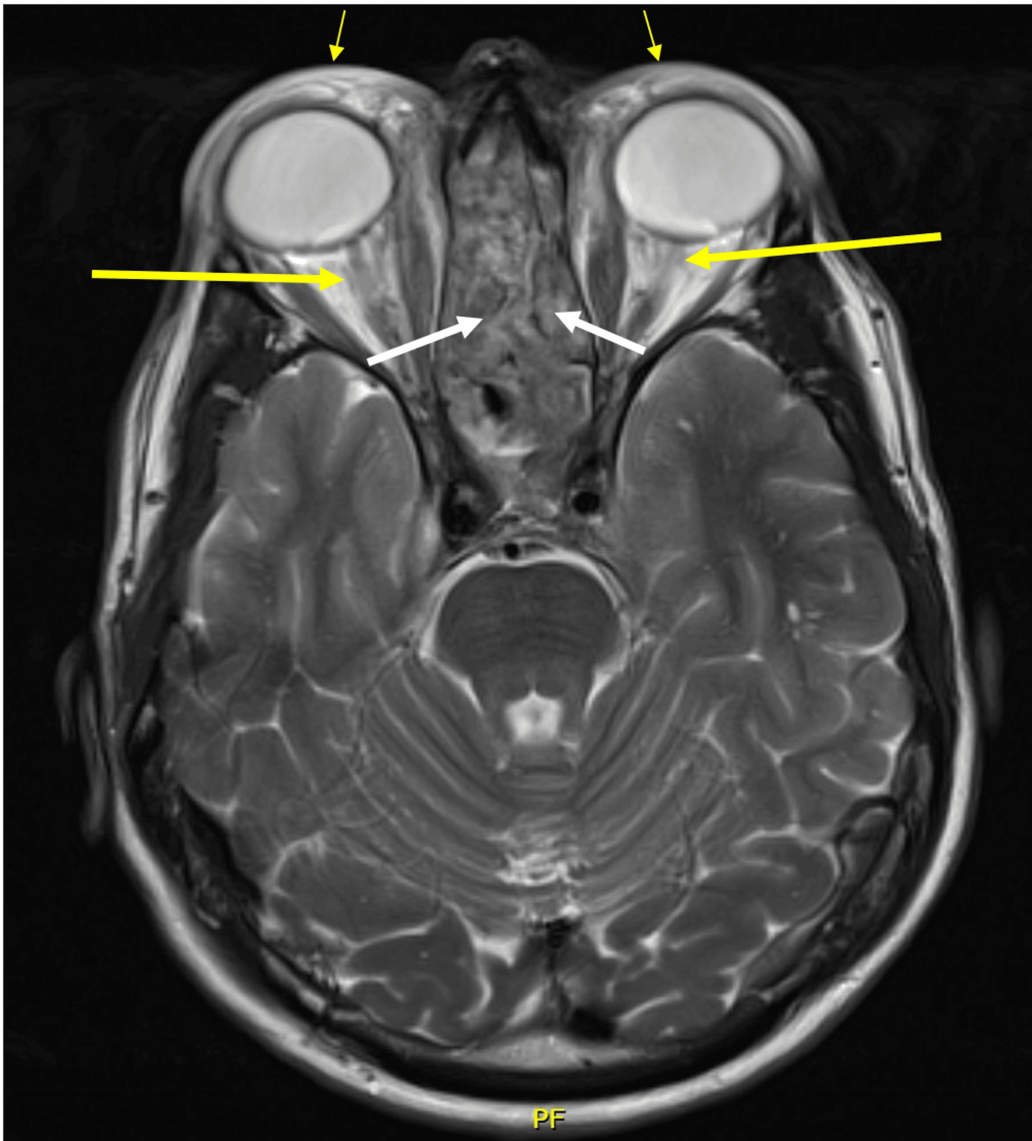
Axial MRI of the orbits MRI (axial view) of the orbits showed bilateral preseptal edema (thin yellow arrows), orbital edema (thick yellow arrows), and pan-sinusitis (white arrows).
